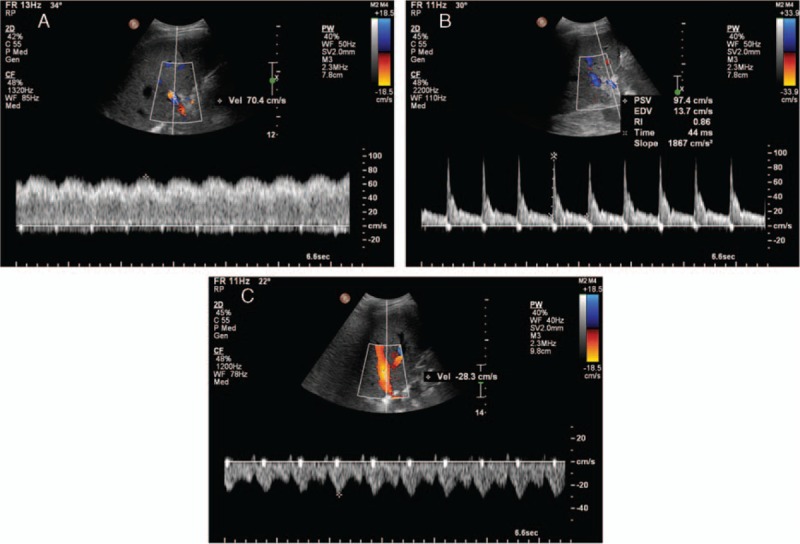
Measurement of PVV (A), PSV and EDV (B), and HVV (C) using spectral Doppler ultrasonography 1 day after living donor liver transplantation. RI is calculated by (PSV–EDV)/PSV. EDV = end diastolic velocity of the hepatic artery, HVV = peak hepatic venous flow velocity, PSV = peak systolic velocity of the hepatic artery, PVV = peak portal venous flow velocity, RI = resistive index.
